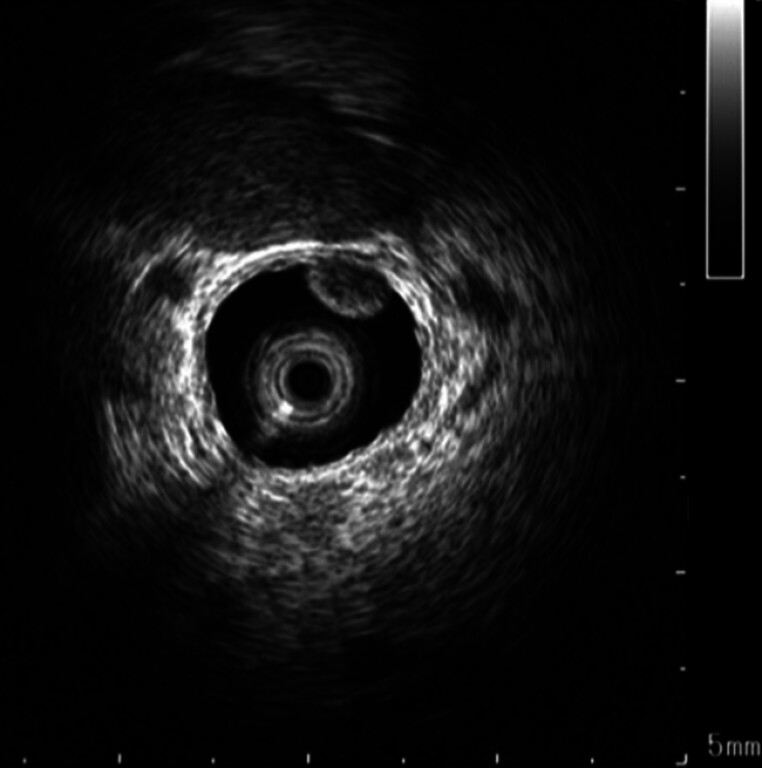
Intraductal ultrasound showed multiple hypoechoic, subepithelial lesion-like protrusions with hyperechoic margins in the right hepatic duct.
